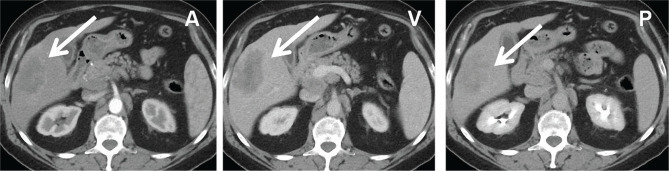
CT demonstrating large liver tumor, hilar adenopathies that cause biliary obstruction and multiple metastatic spinal lesions A – arterial phase; V – venous phase; P – Portal phase.
